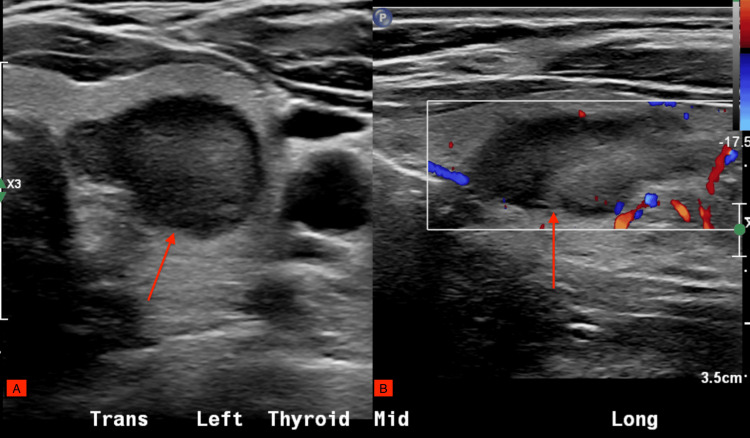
Thyroid ultrasound (24 mm left mid-gland nodule) (A) Trans Mid Left Thyroid. (B) Long Mid Left Thyroid.
